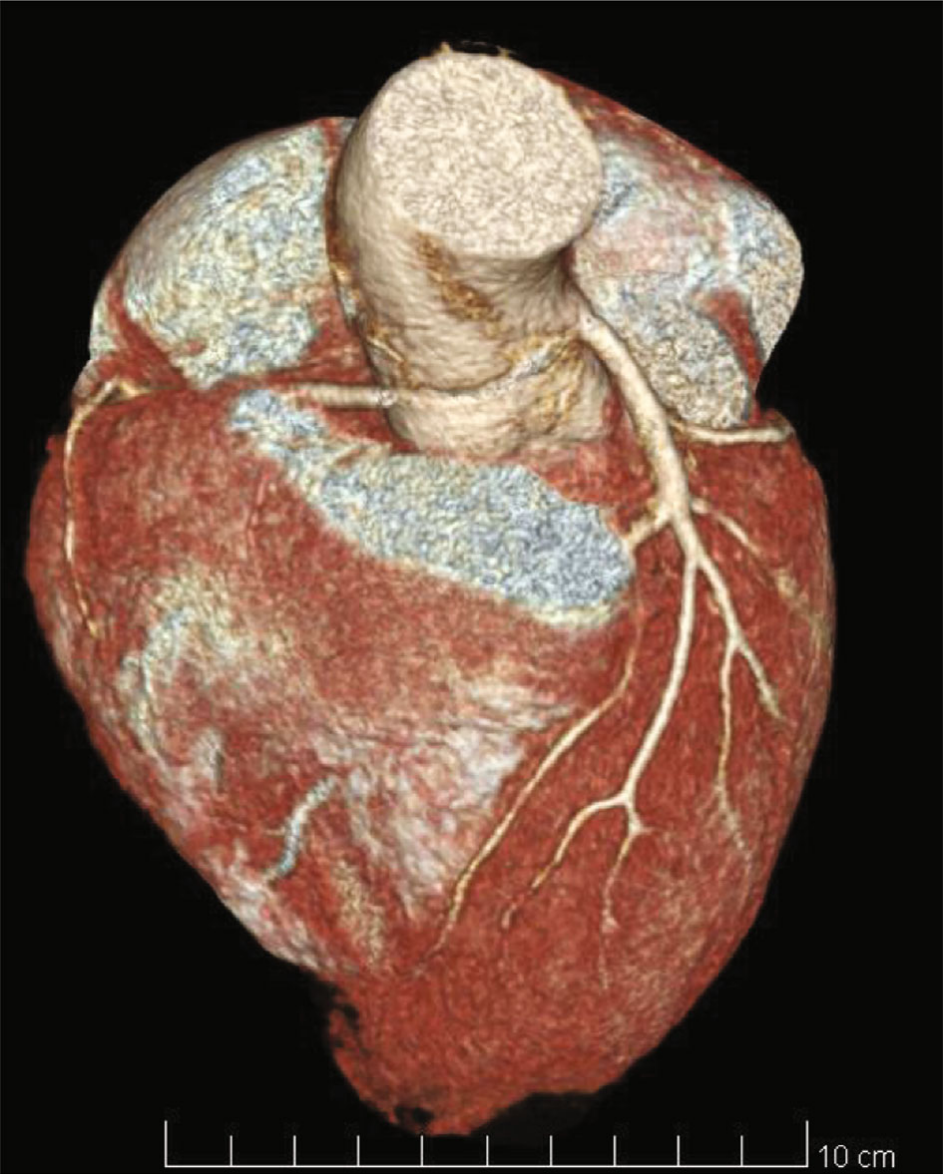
Coronary computed tomography showing the intramural path of the right coronary artery with origin close to the left coronary artery.
